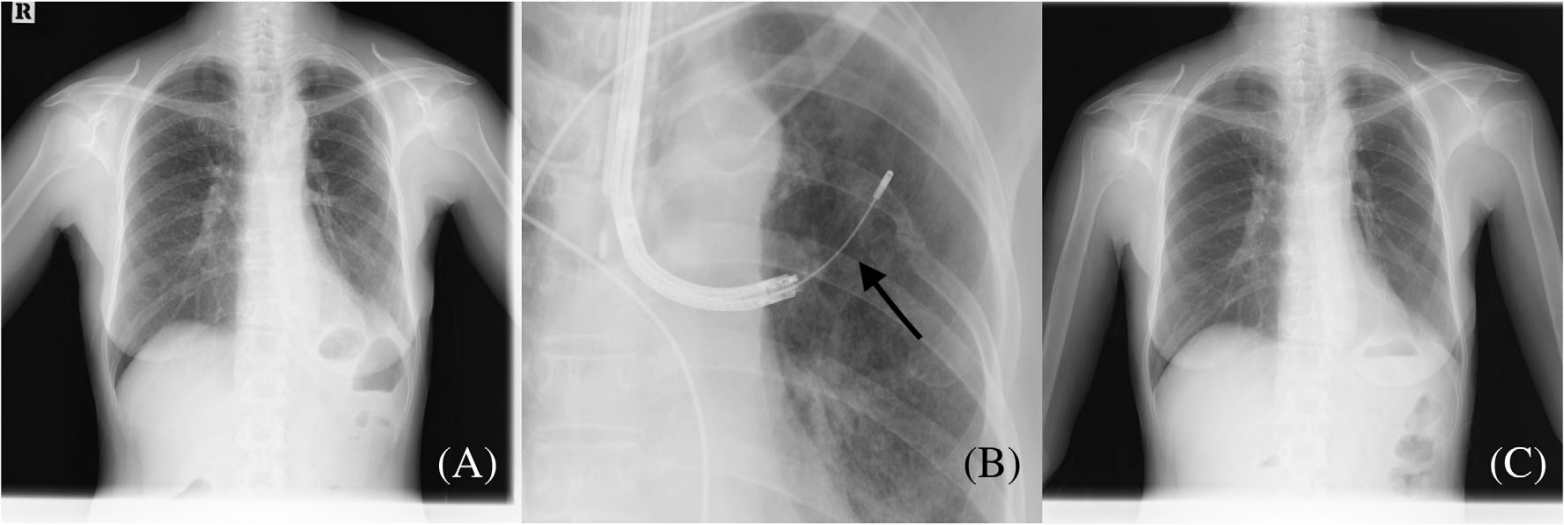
(a) Chest radiograph showed the presence of miliary nodules throughout both lungs. It was later diagnosed as metastatic tumors with L858R and T790M mutations. (b) Transbronchial lung cryobiopsy was performed in the left S6 area. (c) After treatment with osimertinib, the miliary nodules disappeared
